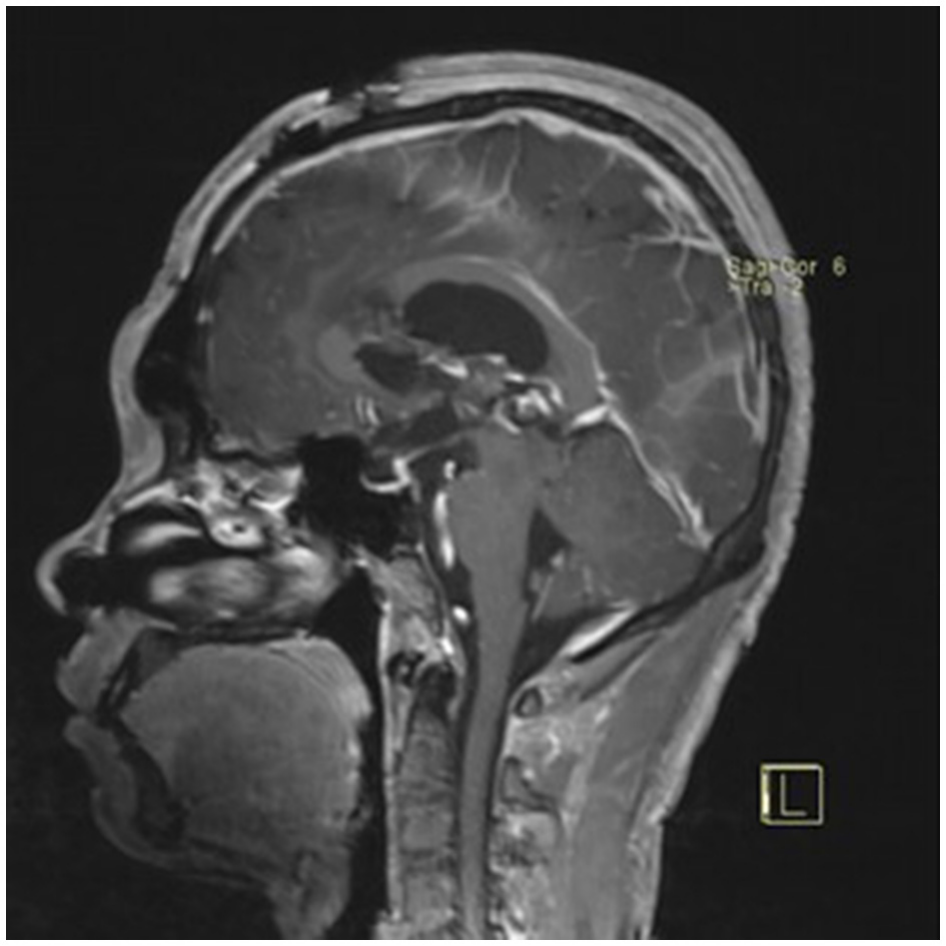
Postoperative contrast-enhanced MRI (sagittal view) showing no residual tumor.
